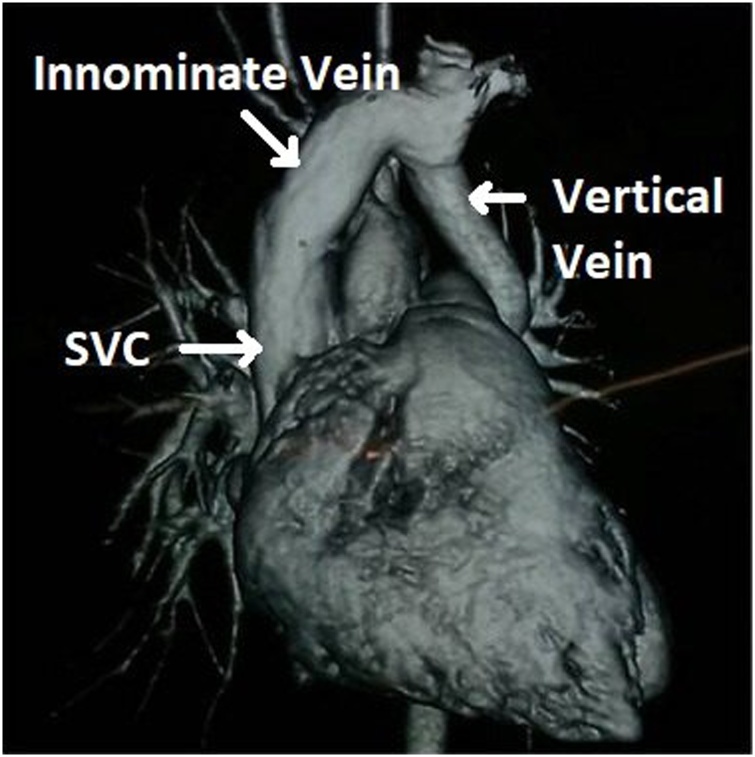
Reconstructed CT Pulmonary Angiography (CTPA) image showing the drainage of the vertical vein into the superior vena cava (SVC) via the innominate vein.
